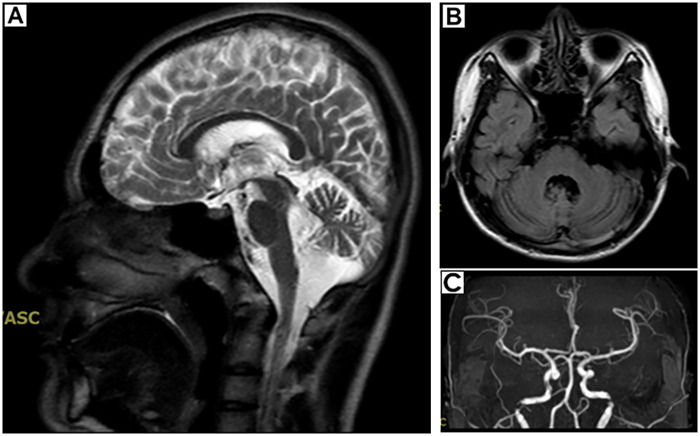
Head MRI. (A) Sagittal plane (B) Transverse plane (C) MRA.
